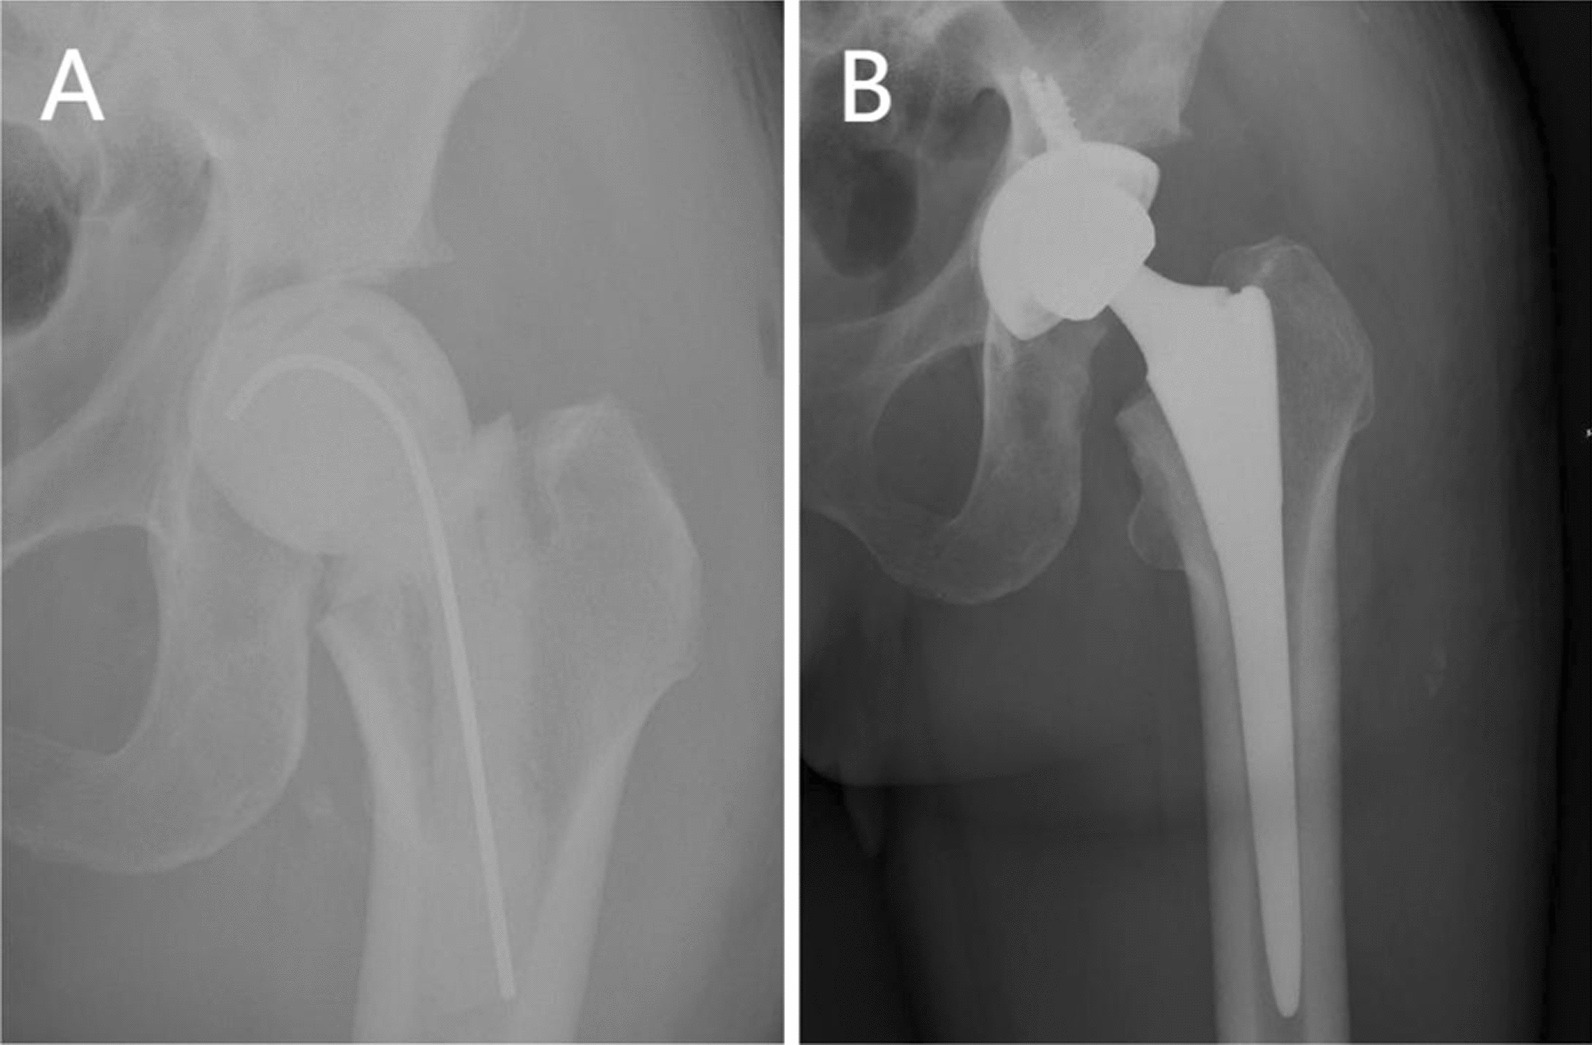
Standard two-stage arthroplasty (case 6). A Radiograph after an antibiotic-loaded cement spacer inserted. B Radiograph after two-stage arthroplasty
